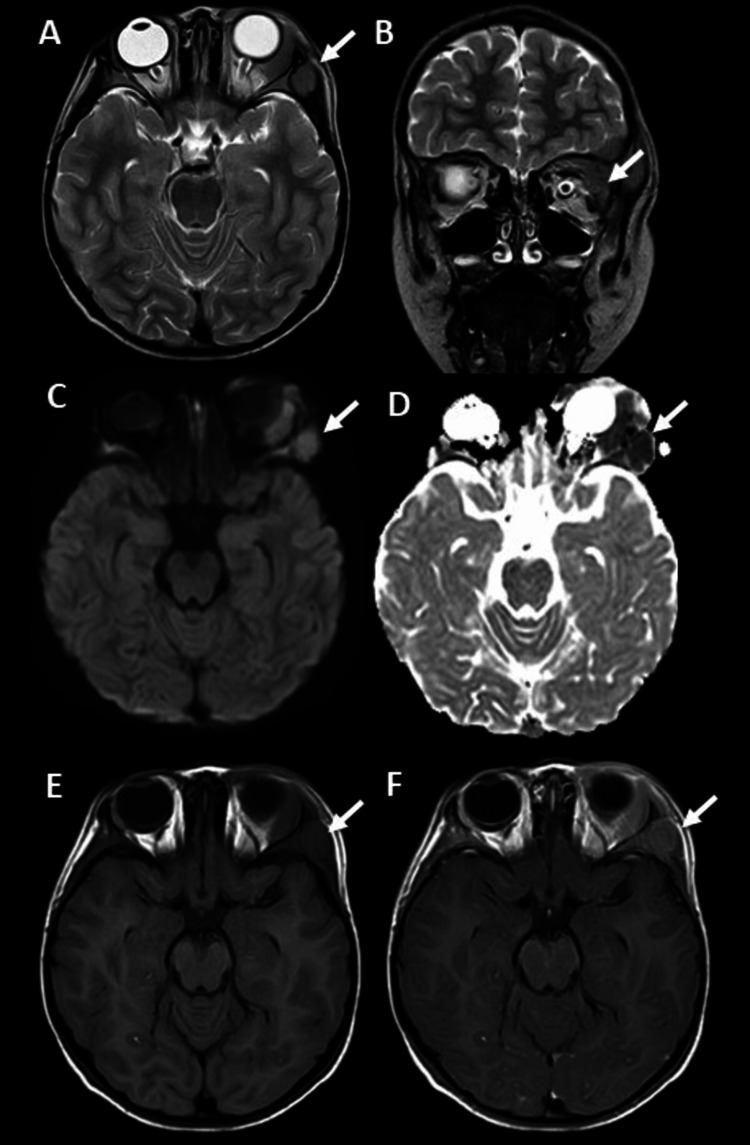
Contrast-enhanced brain MRI in T2-weighted axial and coronal sequences (A and B) showing a hypointense mass in the left frontal bone extending into the extra-axial frontal space, intra and extraconal orbit, and periorbital soft tissues (white arrows). The mass restricts in diffusion sequences, appearing hyperintense in b1000 (white arrow in C) and hypointense in ADC (white arrow in D). It is hypointense in the pre-contrast T1 sequence (white arrow in E), with post-contrast enhancement (white arrow in F). ADC: apparent diffusion coefficient
